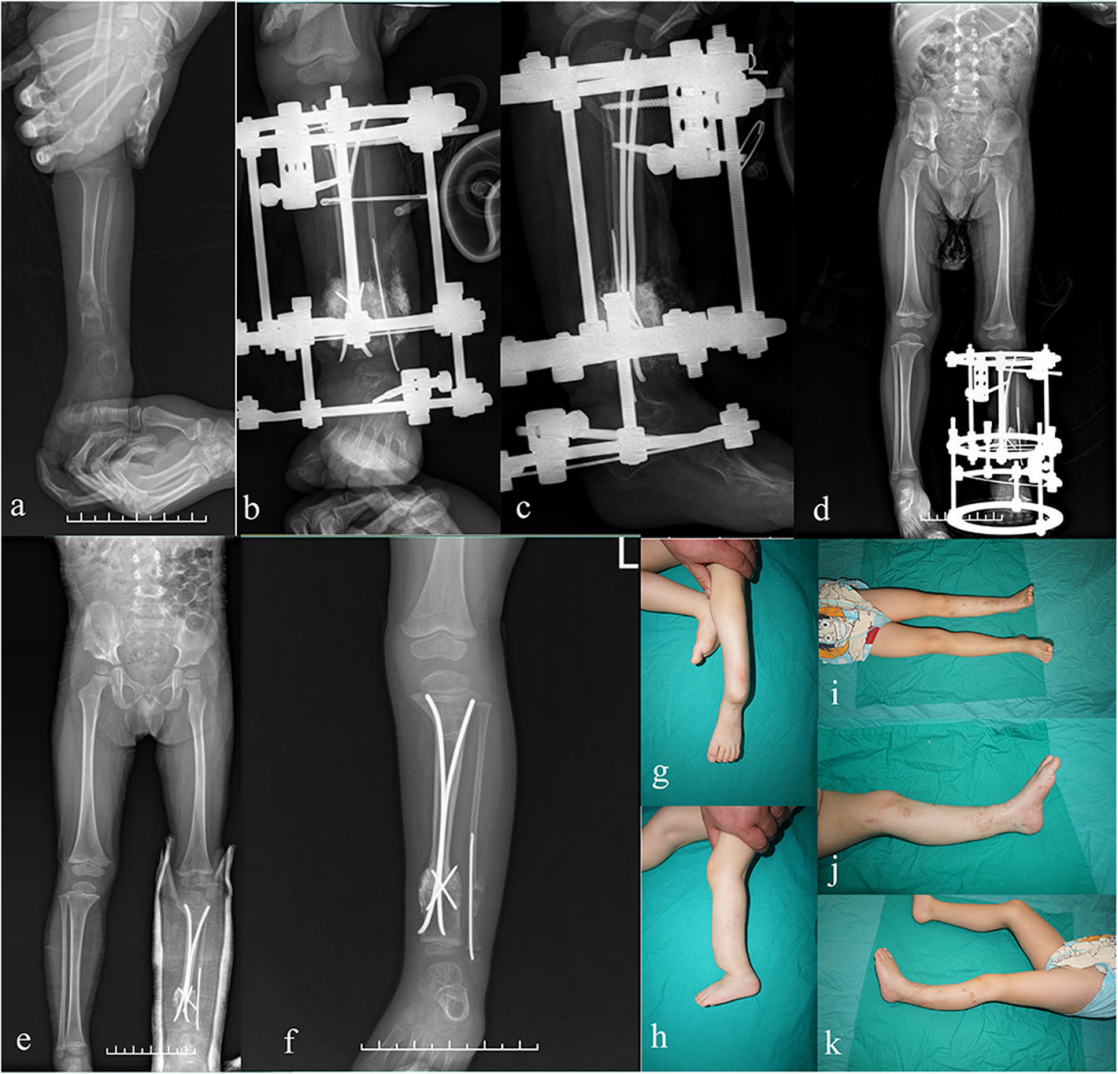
Case 12, a 2.5-year-old boy with CPT treated successfully with pseudarthrosis resection, the “Eiffel Tower” double TENs, bone grafting and external fixation. Pre-operative anteroposterior X-rays showing Crawford type III CPT with anterolateral bowing of tibia (a). Anteroposterior and lateral radiograph of the same patient taken immediately after combined surgery (b, c). X-ray taken 3 months post-operatively shows primary union of the pseudarthrosis site (d). Radiograph taken 6 months post-operatively shows a well-aligned and remodeled tibia without ankle valgus or tibia angulation (e). The nails were retained in the tibia medullary cavity with growth (f). Clinical appearance of the patient before (g, h) and after (i, j, k) the surgery. The patient had an AOFAS score of 82 and a 20 mm leg-length shortening at the finally follow-up
